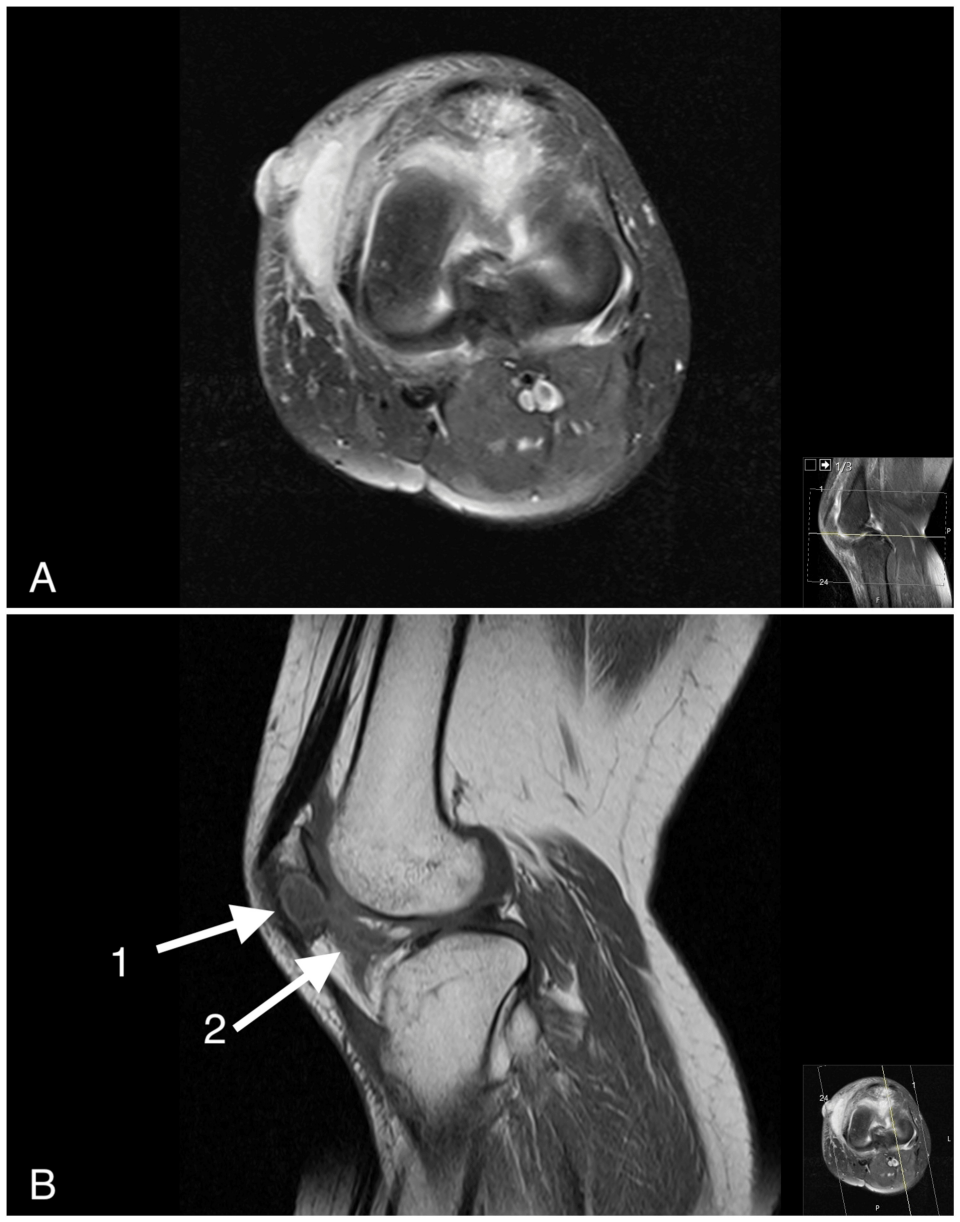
MRI of the left knee (A) Axial PD TSE view of the knee. The high signal highlights the extent of the intra-osseous collection in the patella, which is connected to the sinus tract through Hoffa's fat pad. (B) T1 sagittal view of the knee: (1) the intra-patellar lytic lesion, (2) extension into Hoffa's fat pad
